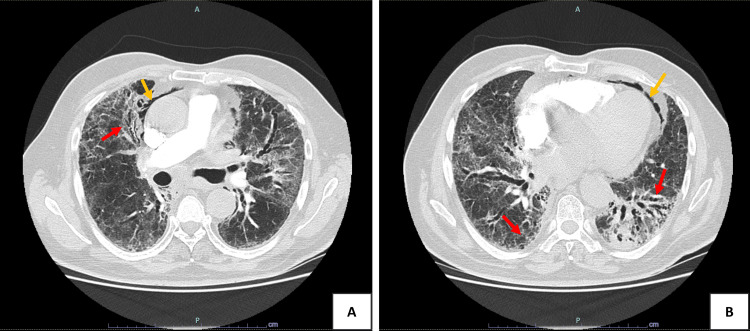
Axial chest CT scans obtained at hospital admission (two months after immune checkpoint inhibitor discontinuation and five months after radiotherapy) (A) and (B) show progression of interstitial involvement, with increased areas of fibrosis and architectural distortion. There is a slight reduction of previous ground-glass opacity, replaced in the same regions by traction bronchiectasis (airway dilatation caused by fibrotic lung remodelling) and parenchymal reticulation of fibrotic character (red arrows). A mild pneumomediastinum is also observed (yellow arrows).
